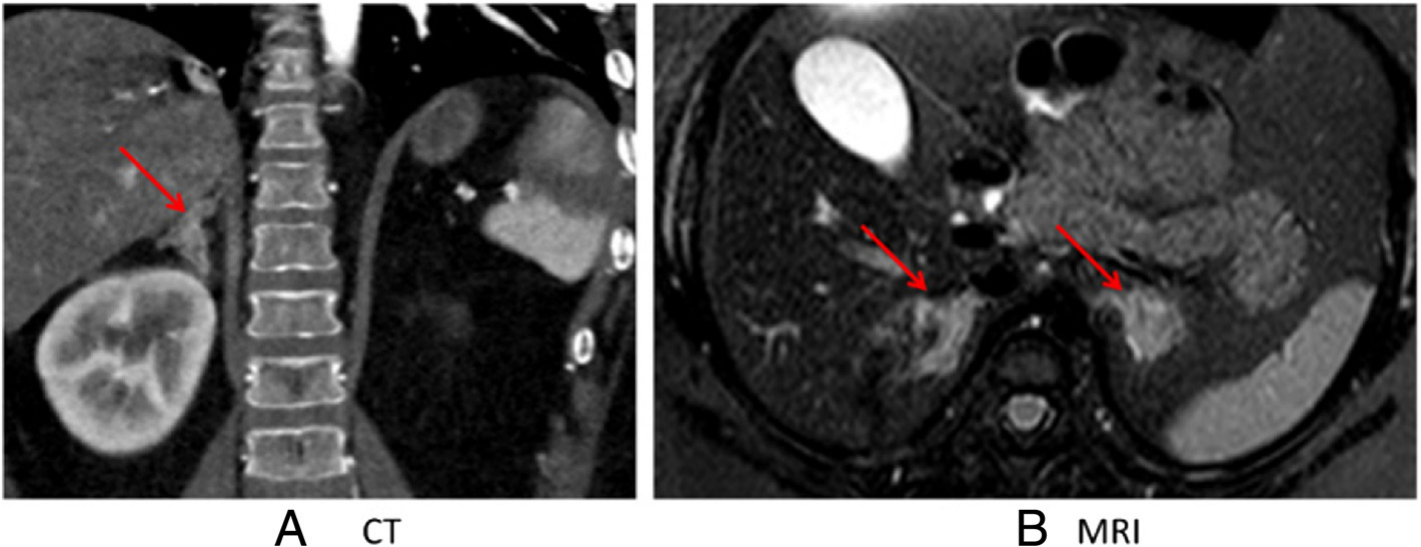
a CT angiogram of the abdomen demonstrating thickened limbs of the adrenal glands with nodular contour. Arrows point to adrenal glands. b Magnetic resonance imaging (MRI) of the abdomen confirmed the presence of thickened nodular adrenal glands with no evidence of a dominant nodule to suggest adenoma or adrenal cortical carcinoma. Arrows point to the two adrenal glands
